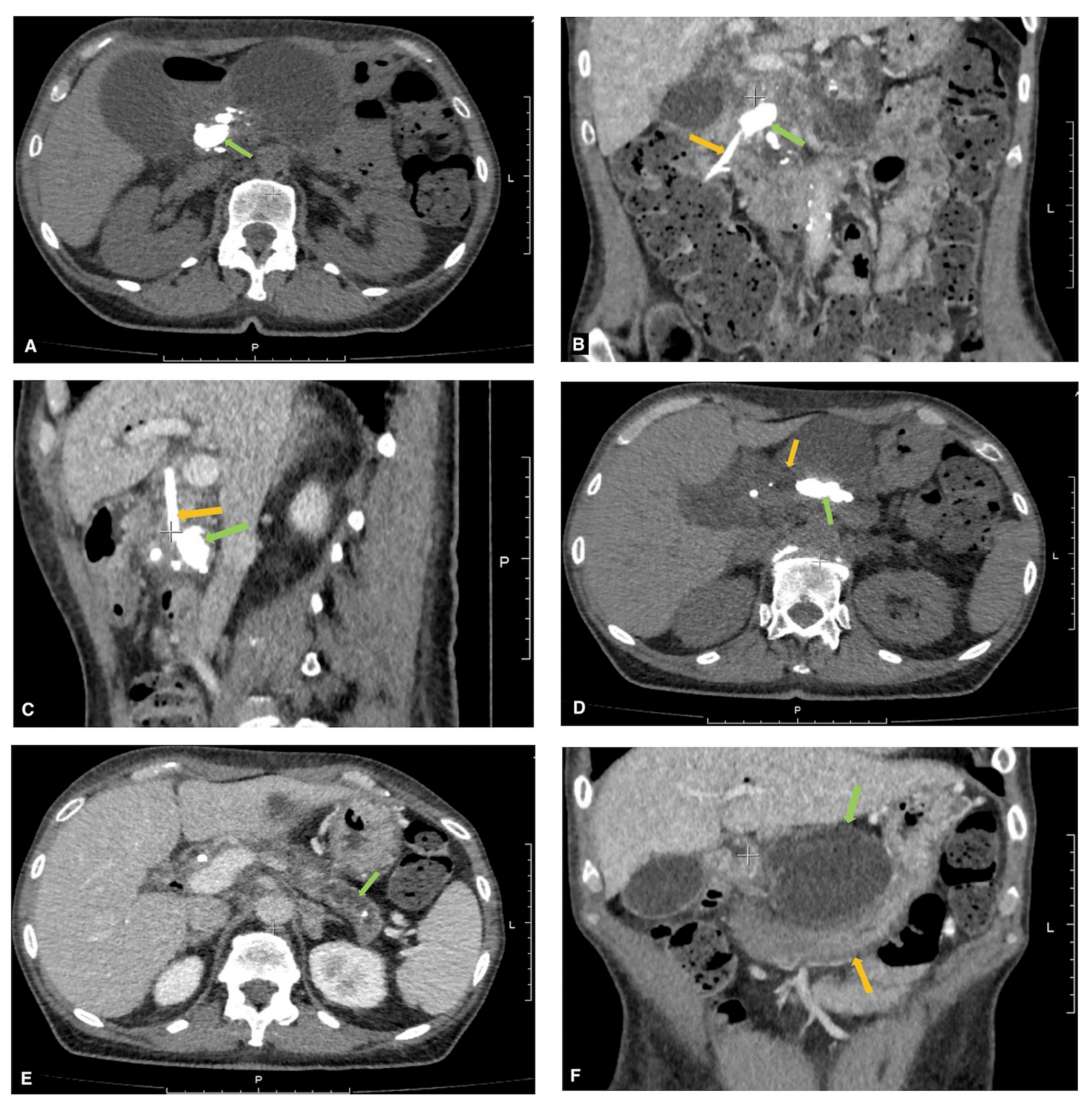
IV contrast-enhanced computed tomography (CT) findings of the abdomen A, B, C) A giant “coral-like” calculus (green arrow) 30 x 35 mm in the head of the pancreas with close contact to the endobiliary stent (orange arrow). D) A giant “coral-like” calculus (green arrow) 32 x 12 mm in the body of the pancreas. The MPD (orange arrow) between the head and body of pancreas is dilated up to 12 mm. E) The MPD (green arrow) distal to the body calculus appears tortuous and dilated (10-12 mm) in the body and tail of the pancreas. F) A pancreatic cyst (green arrow) in the body of the pancreas causing gastric compression (orange arrow).
